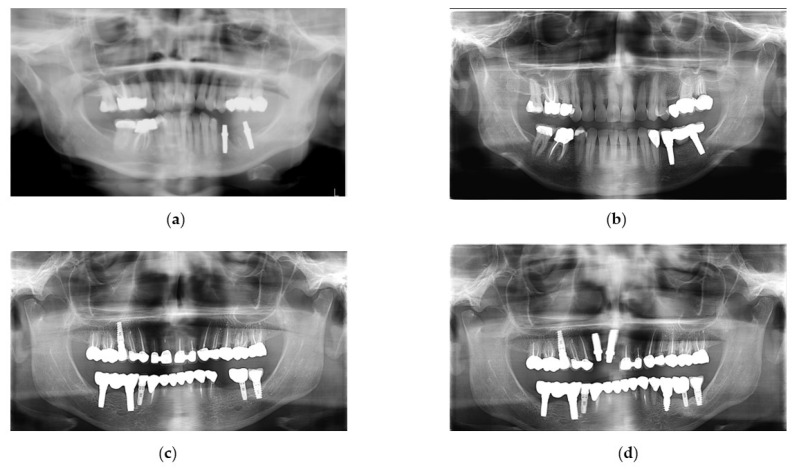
Panoramic radiographs of two patients: (a,b) 73-month follow-up of two O-PZIs supporting FDPs in the third quadrant; (c,d) 51-month follow-up of two O-PZIs supporting FDPs in the fourth quadrant and 23-month follow-up of one O-PZI supporting an SC in the third quadrant.
